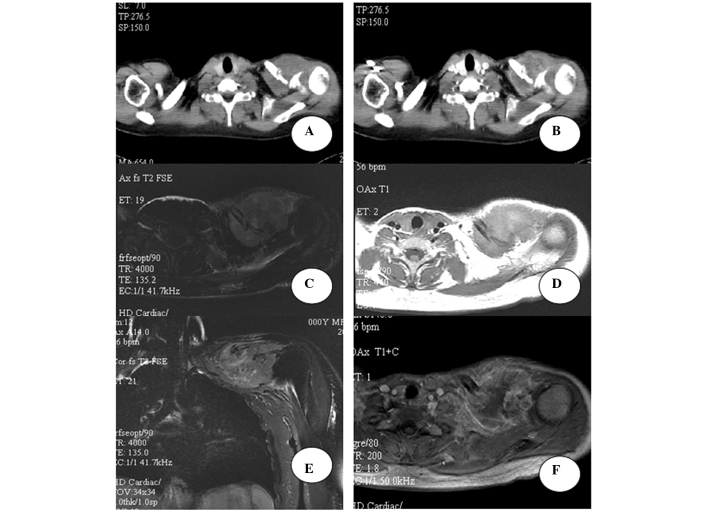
A 27-year-old female with synovial sarcoma. (A–B) Computed tomography revealing a poorly-defined, low-intensity signal tumor mass near the shoulder joint. A contrast-enhanced scan revealing heterogeneous enhancement with osseous destruction of the adjacent clavicle. (C–F) Magnetic resonance imaging revealing a slightly higher-intensity signal relative to muscle on T1-weighted imaging (T1WI) and T2WI. High- and low-intensity signals are also present. Contrast-enhanced scan reveals heterogeneous enhancement with osseous destruction and pathological fracture in the adjacent clavicle.
